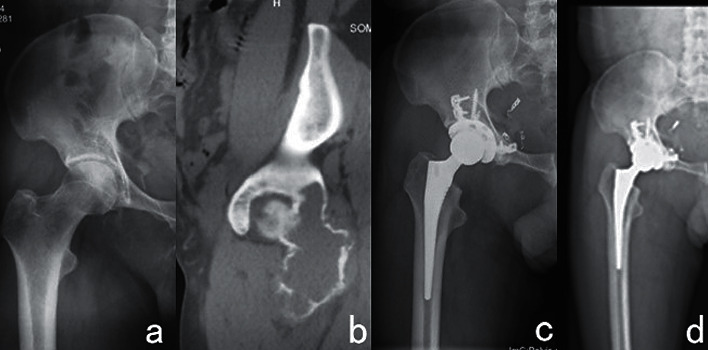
A 44-year-old man with GCT of bone in sections II and III of the right pelvis underwent tumor resection, acetabular reconstruction, total hip replacement, autogenous femoral head, acetabular bone graft, plate and screw fixation, sciatic nerve exploration, and muscle origin reconstruction. (a) Preoperative X-ray. (b) Preoperative CT scan. (c) X-ray at 6 months after the operation. (d) X-ray at 12 months after the operation.
